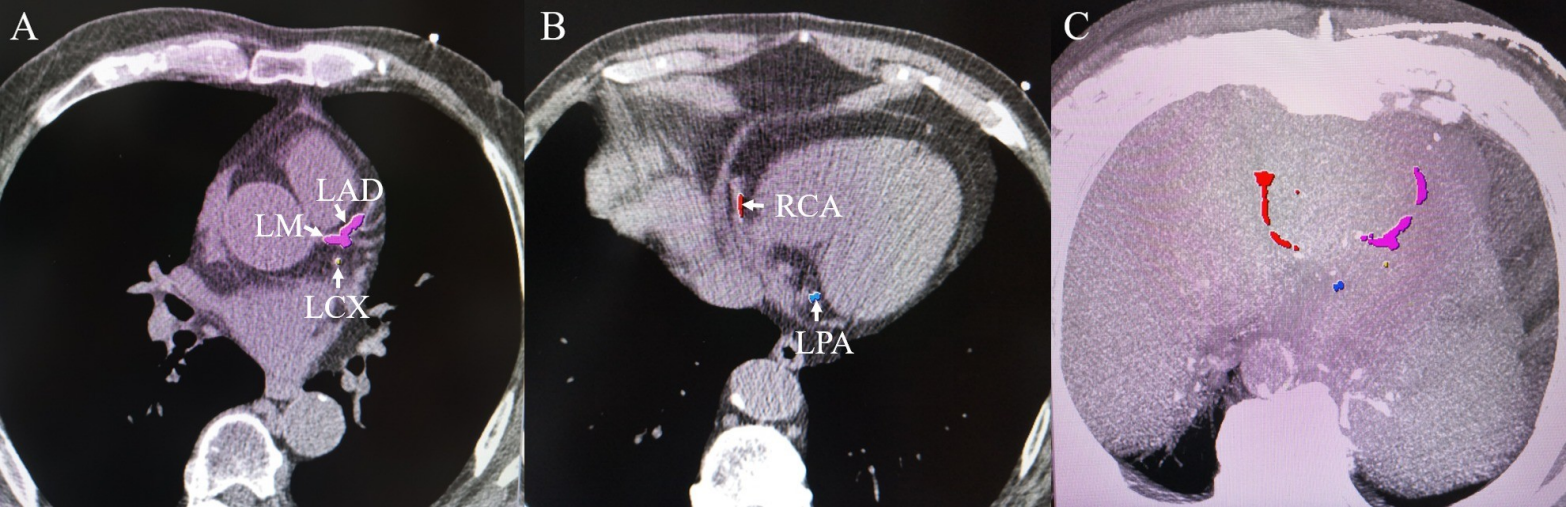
Example of the assessment of the coronary artery calcium score on axial images from the non-contrast CT. (A) and (B) Axial images; (C) superimposed axial images. Extensive calcification observed in the left main coronary artery (LM, pink), left anterior descending artery (LAD, pink), left circumflex coronary artery (LCX, yellow), right coronary artery (RCA, red), and posterior branch of the left ventricle artery (LPA, blue).
